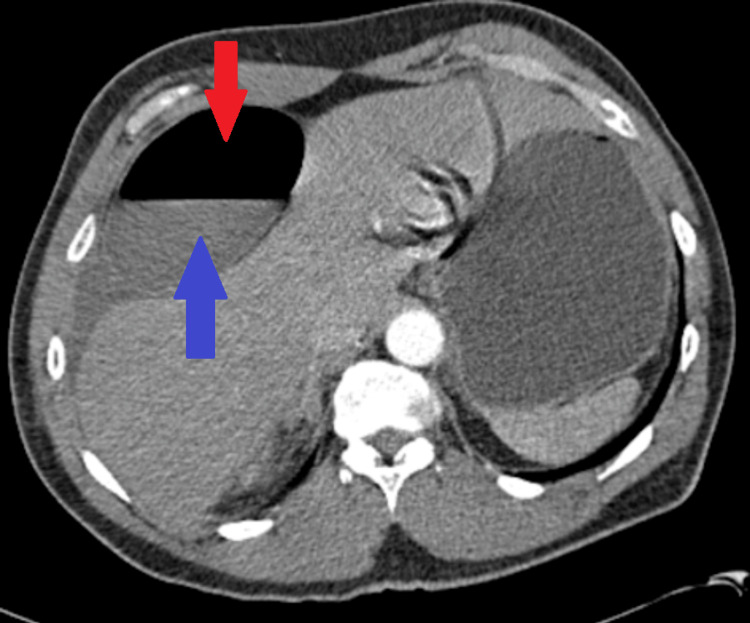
Initial CT angiography with contrast, axial view. The portion marked with the red arrow demonstrates a large intraluminal gas collection, suggesting a segment of bowel or gas within a cystic structure. The portion marked with the blue arrow shows dependent fluid, consistent with a fluid-fluid level within the same structure. There is a large, well-defined, round to ovoid low-attenuation cystic lesion in the left upper quadrant, measuring several centimeters in maximal diameter. The lesion demonstrates homogeneous fluid attenuation without internal septations, calcifications, or enhancing solid components. The wall appears thin and smooth. The lesion exerts significant mass effect as follows: medial displacement of the stomach, posterior displacement of adjacent structures, compression of surrounding bowel loops, and no clear attachment to any other structures. No surrounding inflammatory stranding is seen. No free intraperitoneal air. No appreciable ascites.
